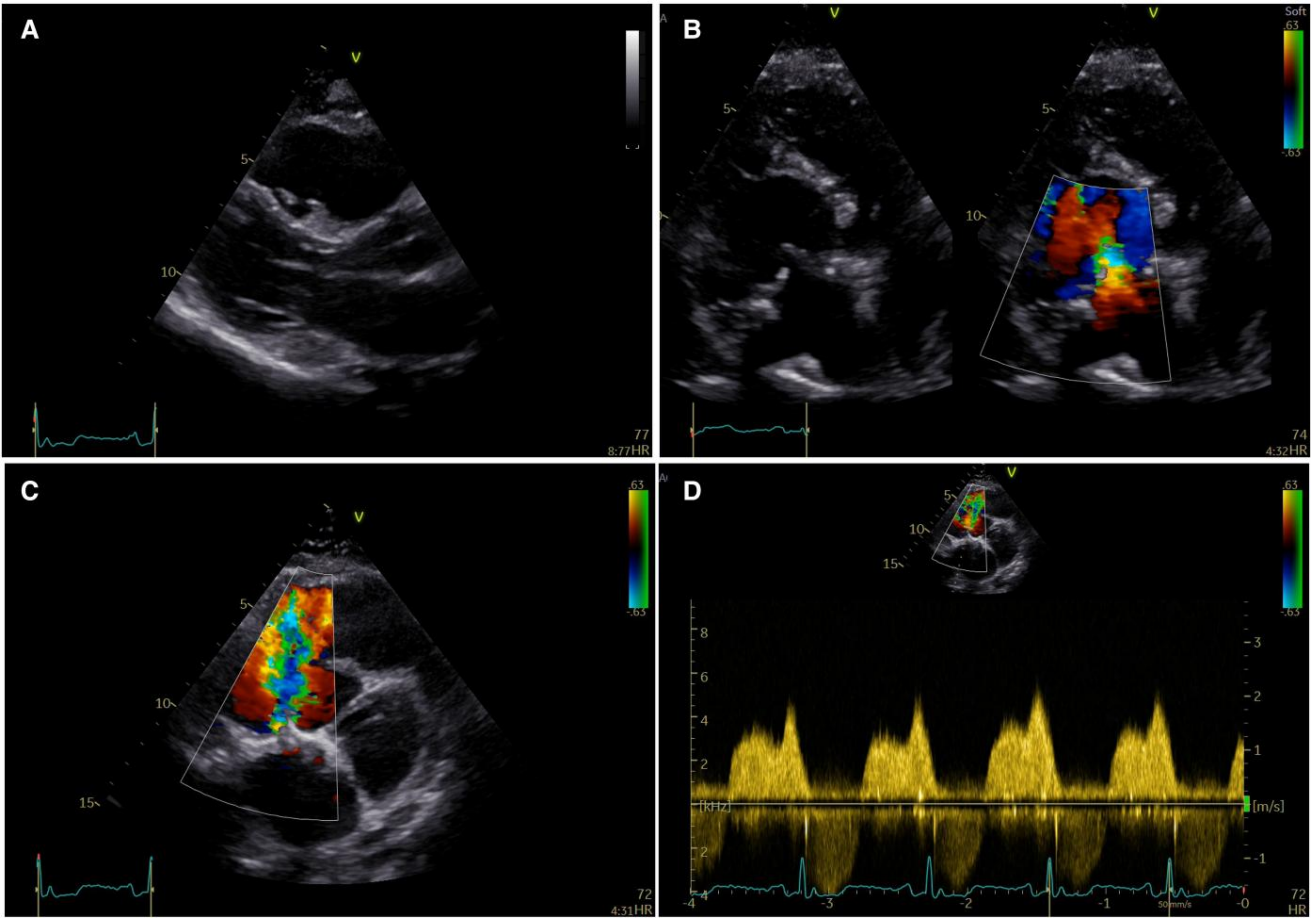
(*A*) Parasternal long axis view by transthoracic echocardiography showing dilated RV with impaired systolic function. (*B*) Apical four-chamber view by 2D transthoracic echocardiography with Doppler imaging with a focus on bioprosthesis at the tricuspid position showing increased flow velocity across the valve. (*C*) Apical four-chamber view with a focus on right ventricular inflow by 2D echocardiography and Doppler imaging showing thickened bioprosthesis valve leaflets with reduced mobility, significant stenosis, and at least moderate regurgitation. (*D*) Continuous wave Doppler imaging showing significant bioprosthesis valve stenosis (mean tricuspid gradient ∼10.9 ± 3.9 mmHg at ∼75 b.p.m.) and at least moderate regurgitation. RV, right ventricle; VSD, ventricular septal defect.
